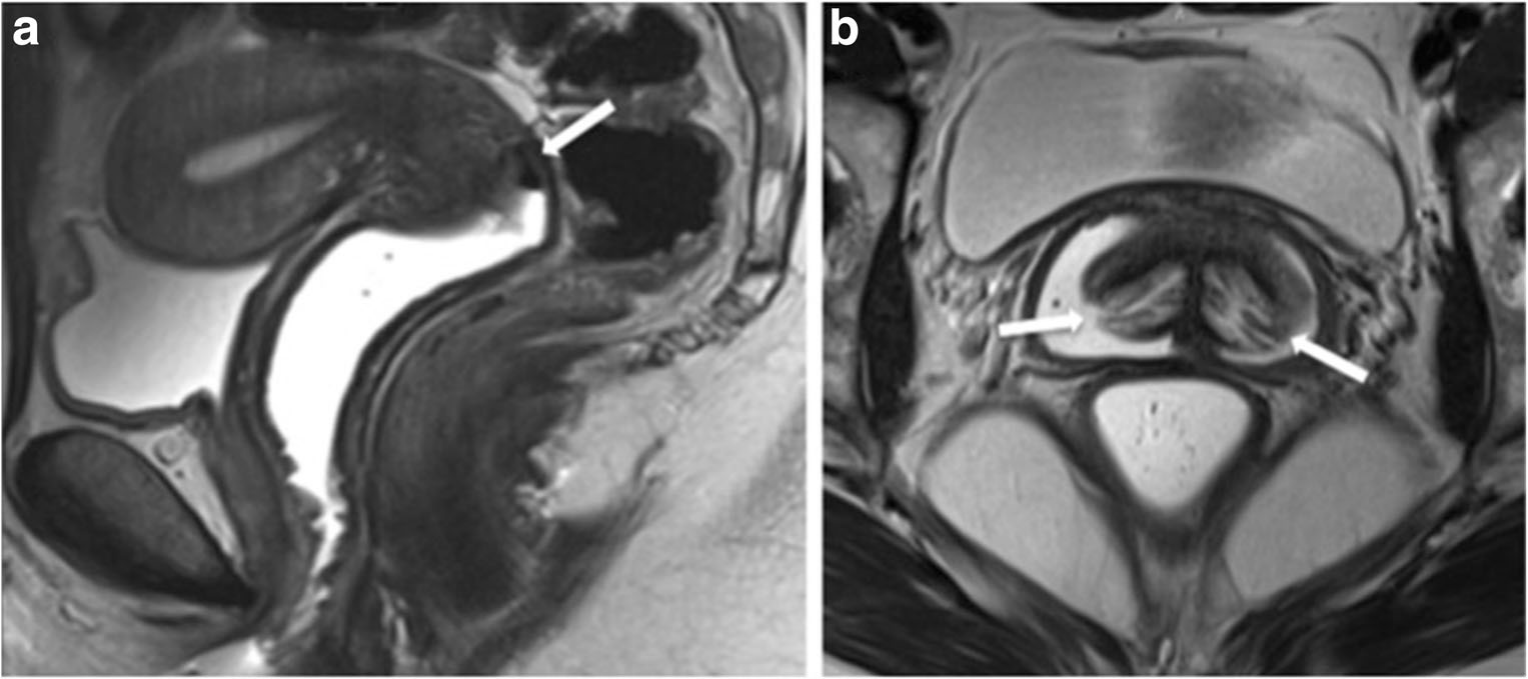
Patient preparation–vaginal opacification. a Self-application of US gel, about 60 mL, in the locker room, just before going to the MRI scanner room. T2W TSE sagittal image (a) showing well-distended vagina, allowing for optimal evaluation. A small amount of air is seen in the posterior vaginal fornix (arrow). The high T2 SI of the gel offers a good contrast with the vaginal walls or a septum (both low SI). b In another patient, with septate uterus with double cervix (arrows), the vaginal gel allows for optimal assessment of the cervical morphology
